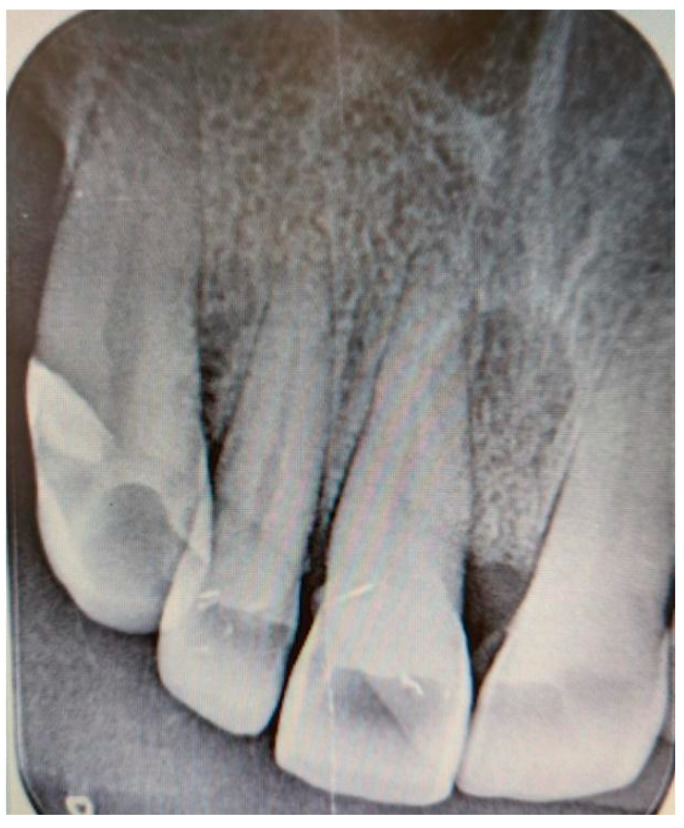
Digital periapical radiograph of the patient’s maxillary incisors.
